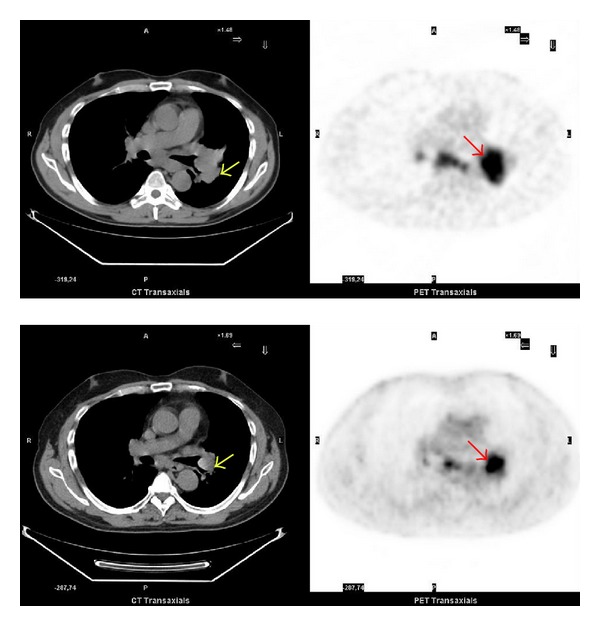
Pre- and posttreatment ^18^F-FDG PET/CT scan. Top: pretreatment ^18^F-FDG PET/CT axial image: staging on a patient with a left hilar adenocarcinoma (yellow arrow) with a SUVmax = 11 (red arrow) and diameter of 40 mm. Abnormal uptake present also in a subcarinal lymph node. Bottom: posttreatment ^18^F-FDG PET/CT axial image: the study was performed one month later, at the end of the first-line chemotherapy. Note the decrease of the ^18^F-FDG uptake (red arrow) in the primary lung lesion (SUVmax = 8) and also an important reduction of the lesion size (yellow arrow).
